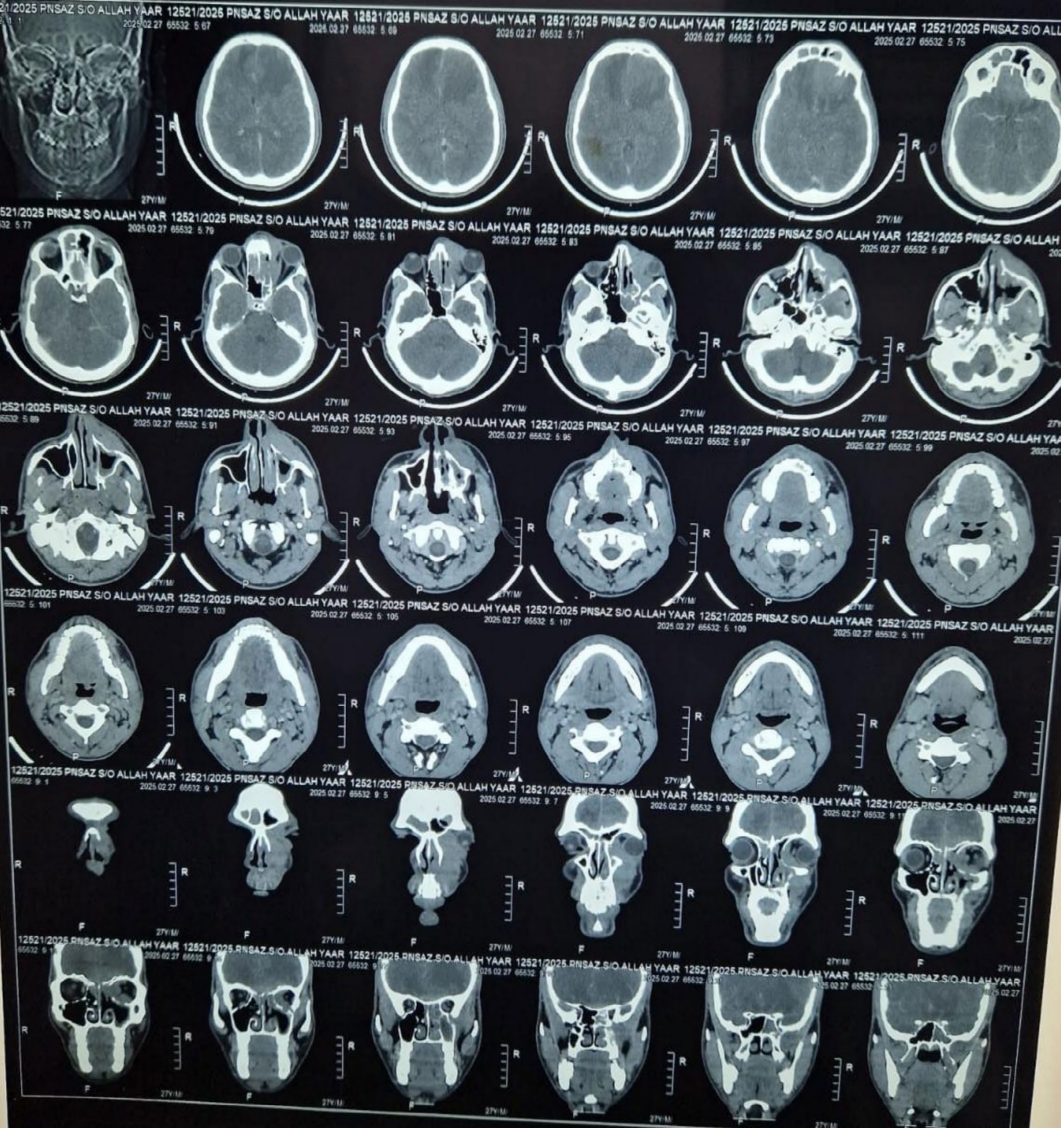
Computed tomography (CT) demonstrating sinonasal and orbital involvement. Axial, non‐contrast computed tomography scan of the paranasal sinuses. The image reveals extensive soft‐tissue density opacification of the left ethmoid and sphenoid sinuses. There is clear evidence of bony erosion, particularly involving the lamina papyracea (the medial wall of the orbit, indicated by the white arrow), signifying invasive disease with extension into the left orbit. Associated inflammatory changes are noted in the adjacent preseptal soft tissues.
